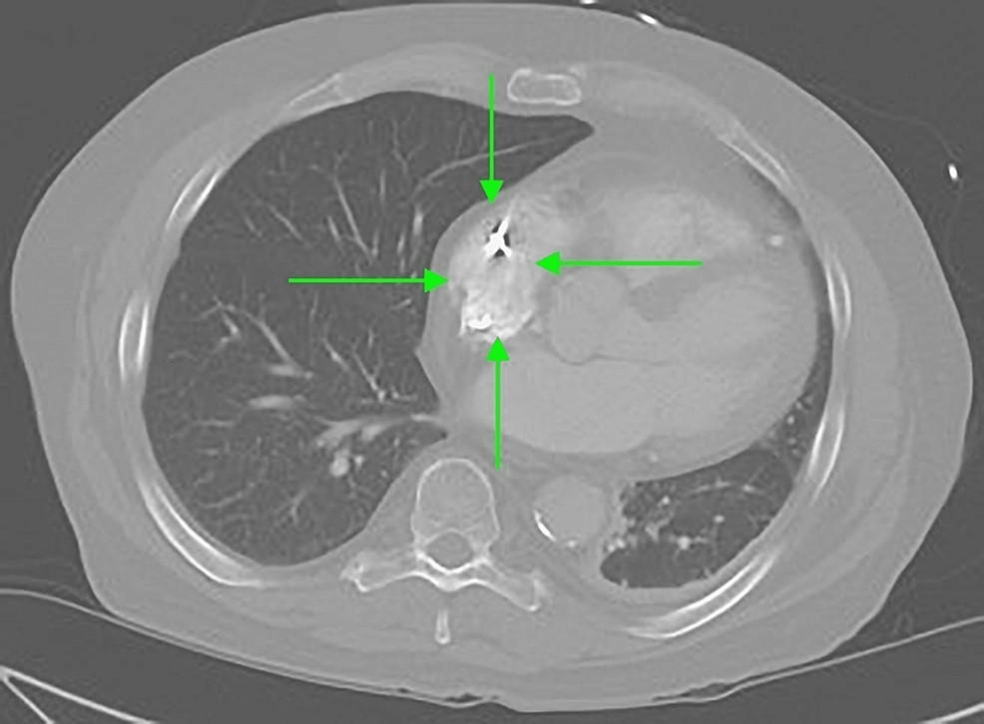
CTA of the chest, horizontal section, revealing small intra-pericardial hernia CTA: computed tomography angiogram.
